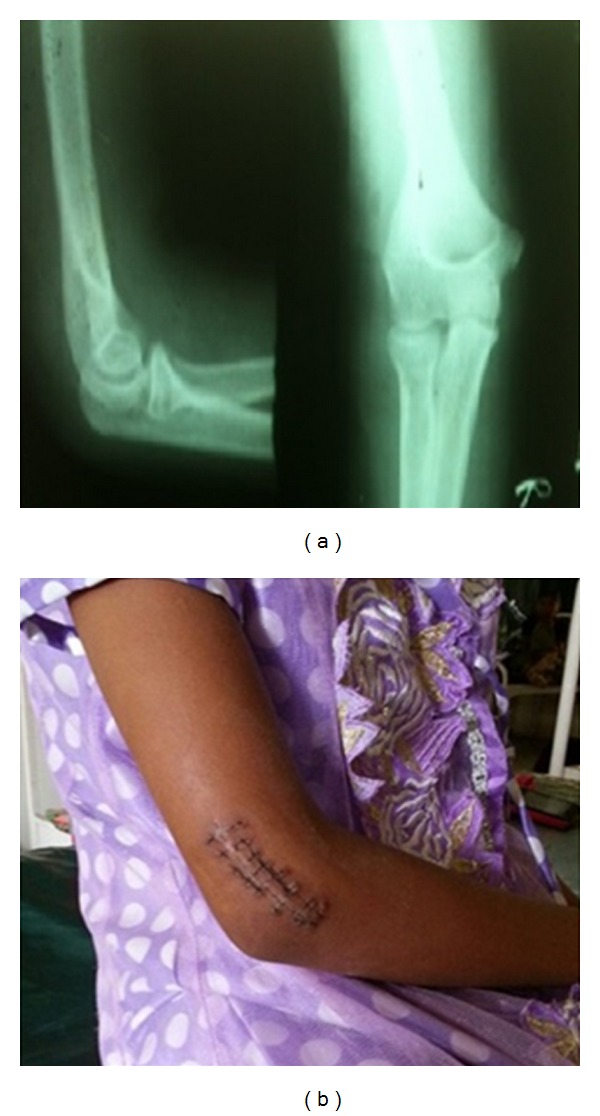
(a) Postoperative radiograph at 1-year follow up showing no evidence of recurrence. (b) Postoperative scar photograph after excision biopsy.
